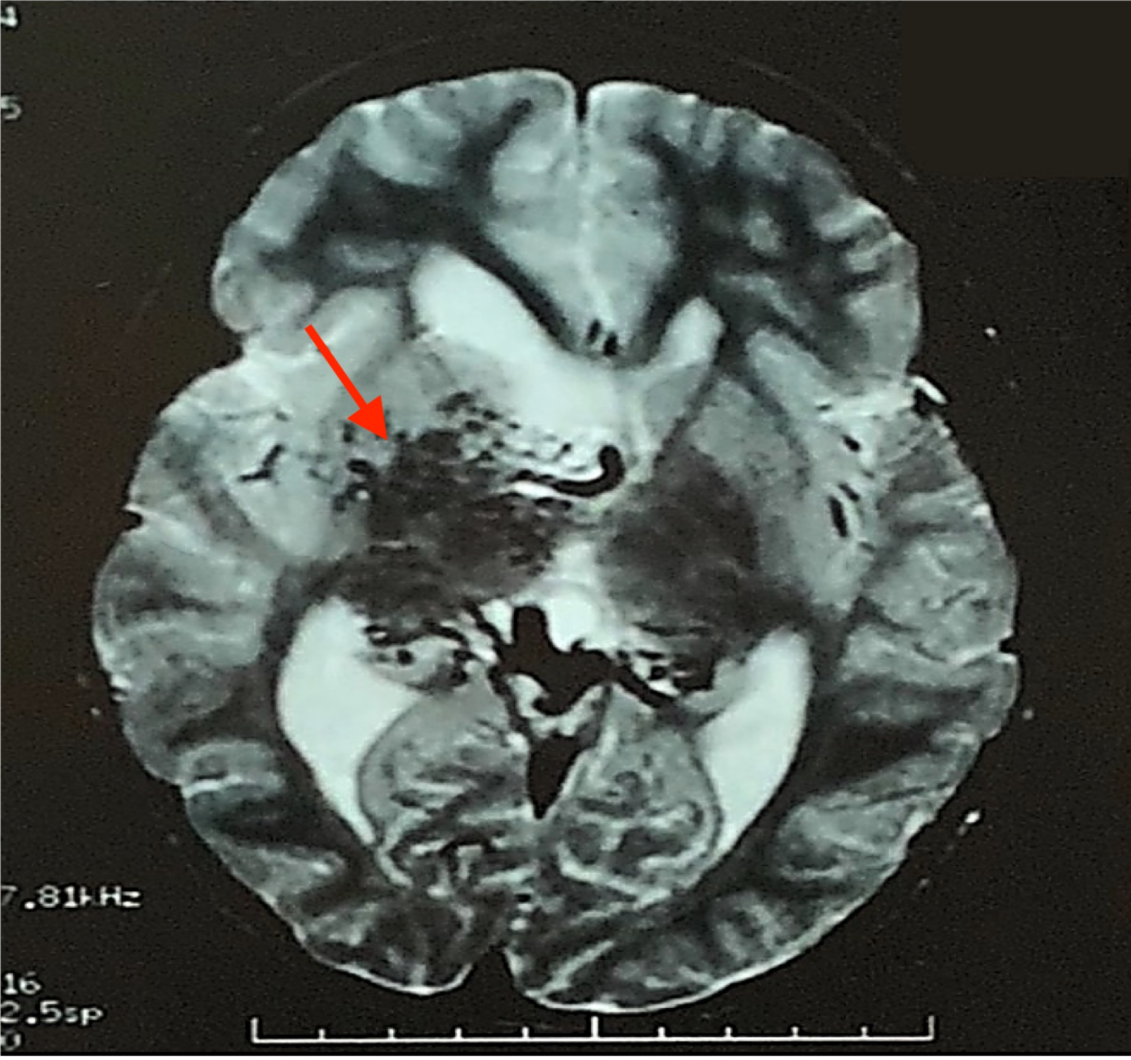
MRI with gadolinium. MRI with gadolinium demonstrating a 5 cm x 4 cm x 3 cm arteriovenous malformation involving mainly the thalamus on the right side and the basal ganglia with large intraventricular draining veins into the galenic venous system.
